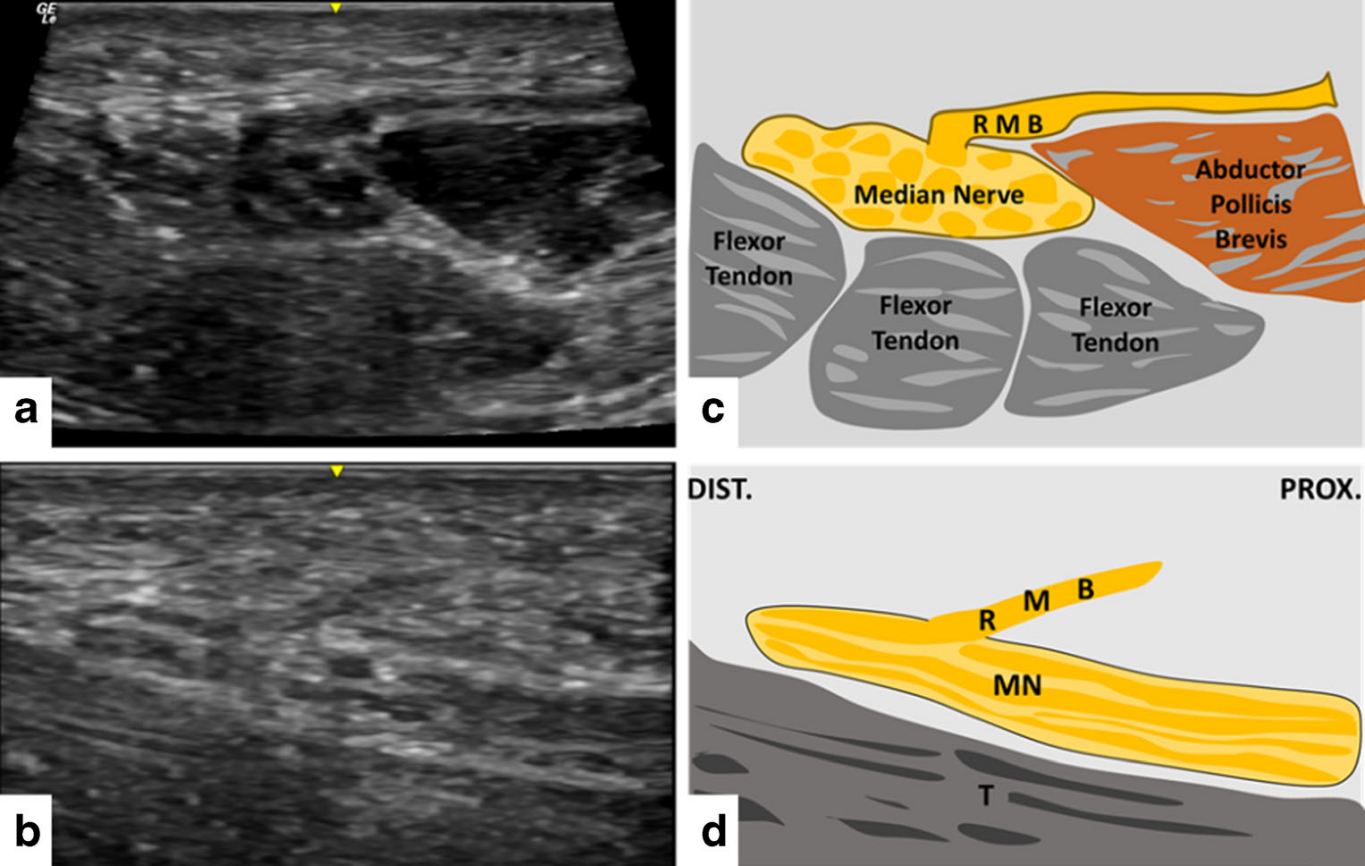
(a, b) Example of sonographic findings of the recurrent motor branch (RMB) at its origin from the central aspect of the median nerve in a radio-ulnar transverse view. (c, d) Example of sonographic findings on a long-axis view of the RMB at its origin from the central aspect of the median nerve coursing proximally toward the thenar. The median nerve and flexor tendons are obliquely projected. Note the approximately 45° angle of the RMB with the median nerve. *FT* flexor tendon, *MN* median nerve, *DIST*. distal, *PROX*. proximal
